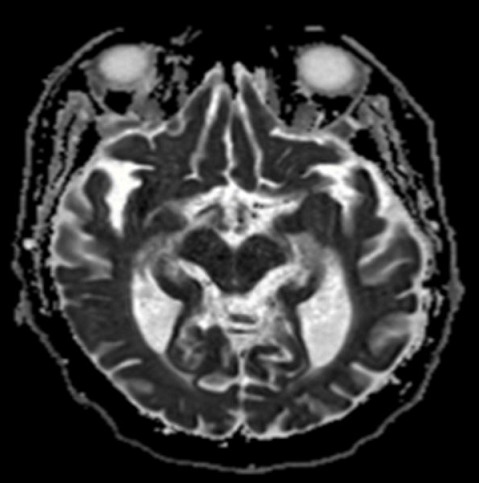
A magnetic resonance imaging (MRI) brain showing a corresponding hypointense signal on apparent diffusion coefficient (ADC) sequence
